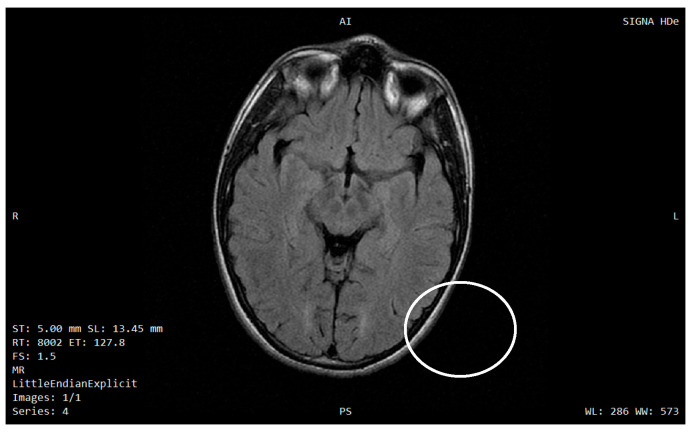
Axial FLAIR MRI showing supratentorial white-matter hyperintensities (circled) in a patient with genetically confirmed Wolfram syndrome, consistent with the neurodegenerative pattern described in this condition. A = anterior; P = posterior; R = right; L = left.
